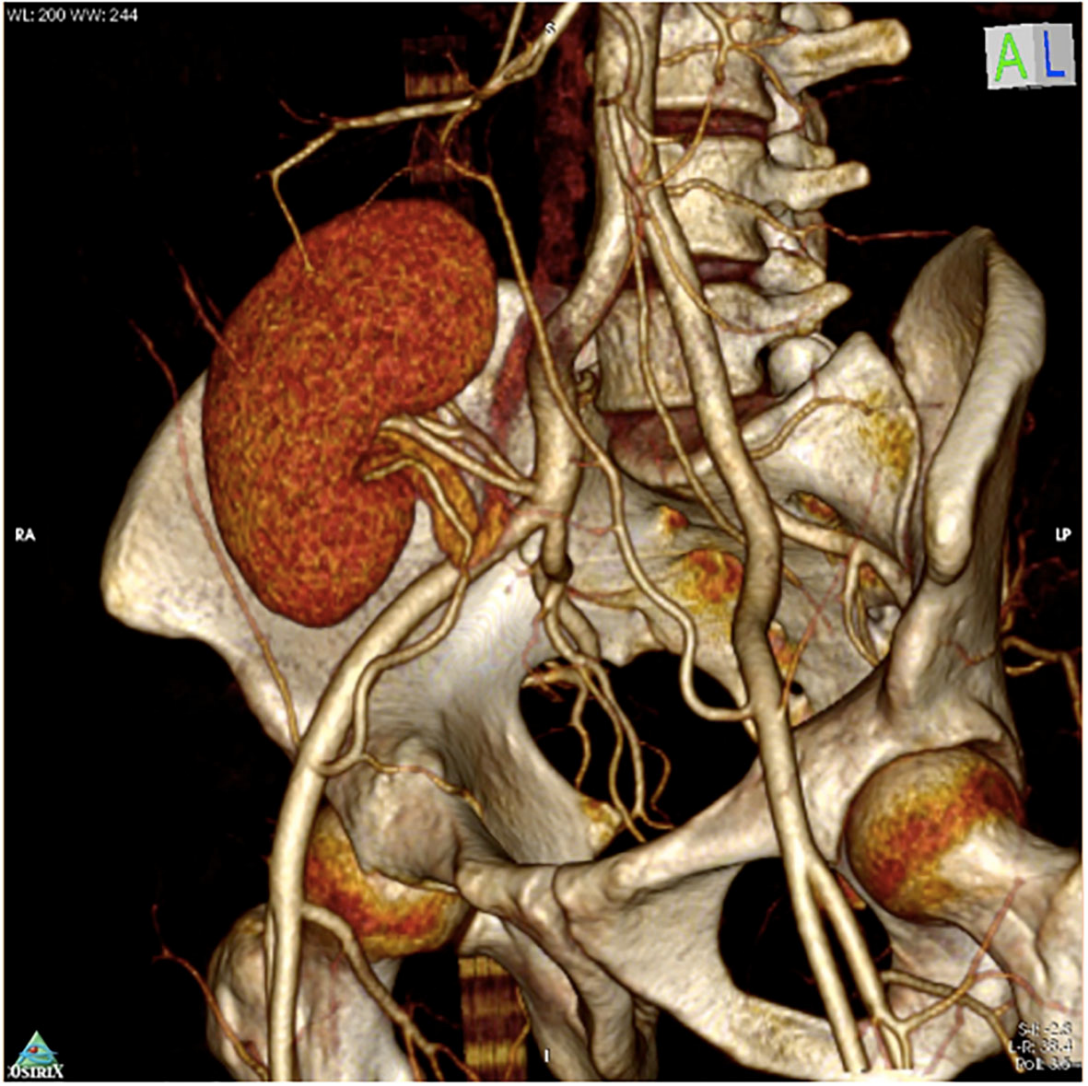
CT angiogram after 3 months after RAKT showing patent Pantaloon and accessory lower pole vessel anastomosis.
